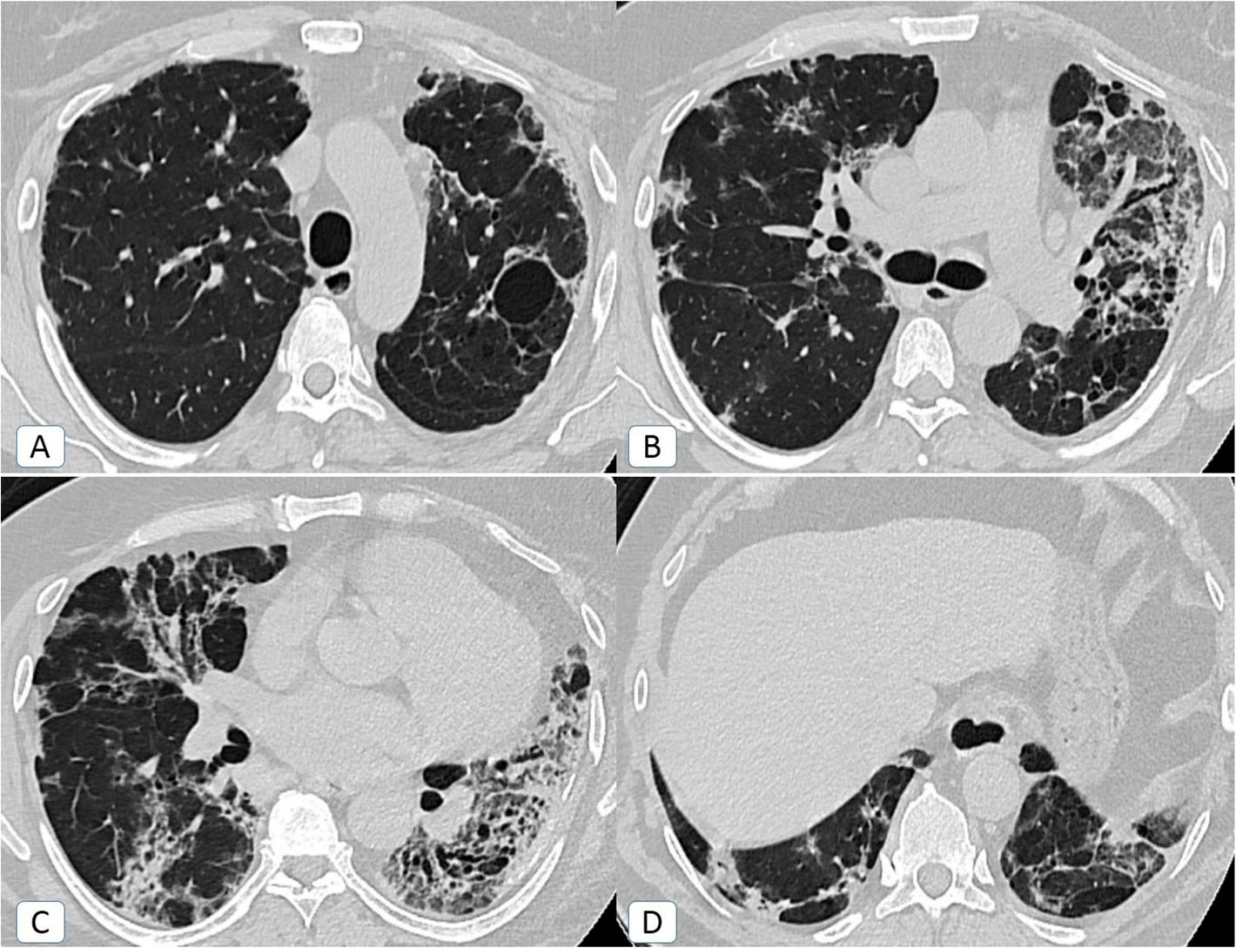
COVID-19 infection on top of old TB and fibro-thorax: a 55-year-old female COVID-19 patient. A. Axial chest CT (lung window) showing bilateral apical pleural and sub-pleural fibrotic changes, more accentuated on the left side with cystic changes and centrilobular emphysema. B, C Axial chest CT (lung window) showing right upper and lower lobar peripheral located ground glass patches of recent COVID-19 infection. This is together with a diminished volume of the left hemithorax which showed ground glass changes, fibrotic changes with traction bronchiectasis and centrilobular emphysema. D Axial chest CT (lung window) showing bilateral basal fibro-atelectatic and curvilinear bands
